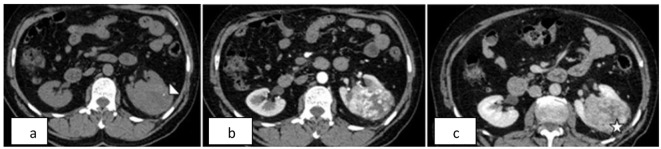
Left renal mass. Non-contrast and contrast-enhanced axial CT of the abdomen shows a well-defined mass in the interpolar region of the left kidney. a) Non-contrast CT shows a small focus of calcification (arrow head) within the mass. b) Arterial phase image shows heterogenous and intense enhancement. c) Venous phase image shows relative washout with areas of necrosis (asterisk) within the mass.
